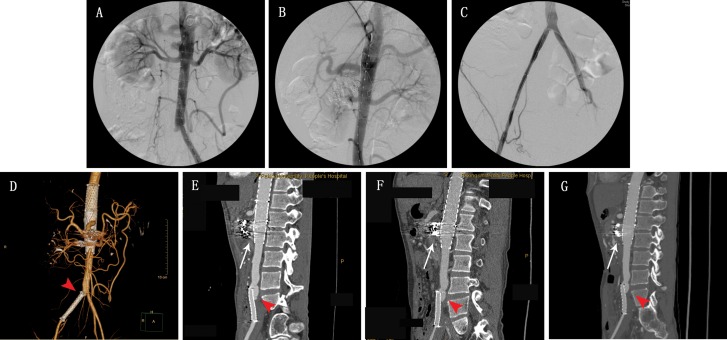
A 42-year-old male with descending thoracic and infrarenal abdominal aortic pseudoaneurysms (A, B). Aortogram following stent graft placement demonstrating that the aneurysm was successfully isolated (C, D). At the 1-week, 2-month, 6-month, and 12-month follow-up exams (E, F, G, H), three-dimensional volume rendering computed tomography (CT) images revealed that the aneurysm had completely regressed.
